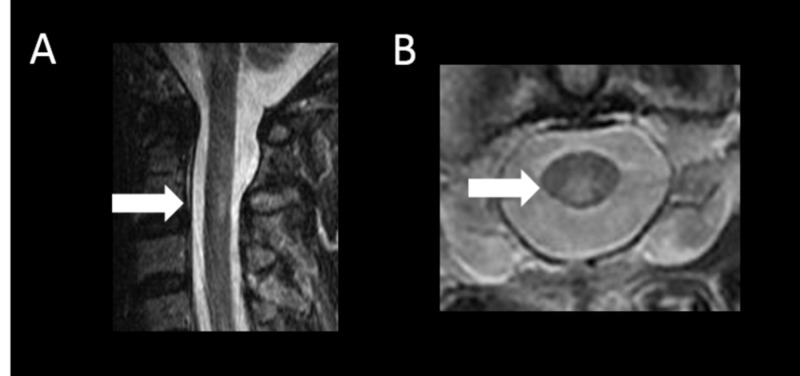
Spinal cord MRI findings A, B: spinal cord T2-weighted imaging shows 11 high-intensity white matter lesions with a length equivalent to less than 1.5 vertebral bodies (white arrows) MRI: magnetic resonance imaging
